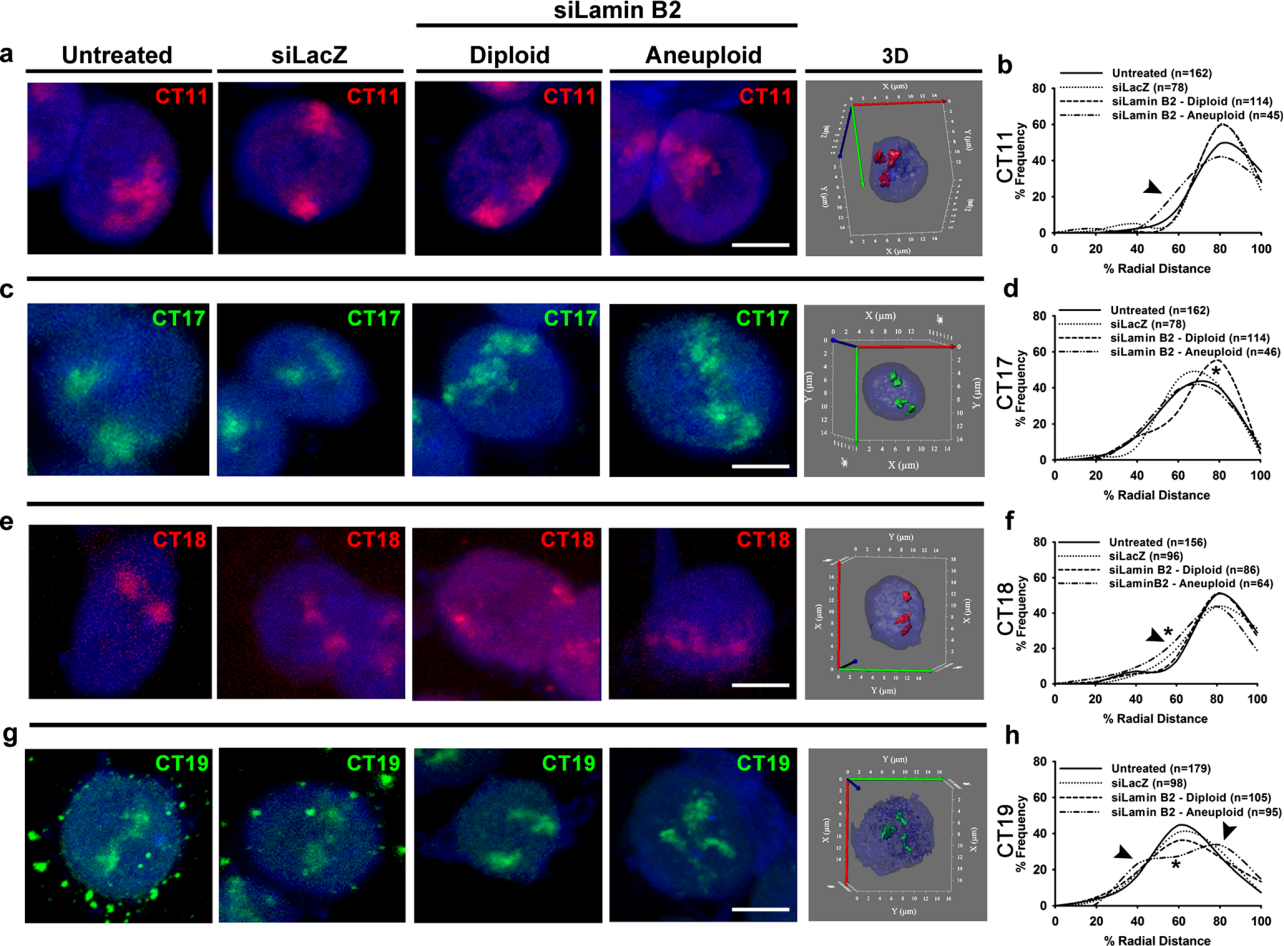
Aneuploid chromosome territories are mislocalized upon Lamin B2 depletion. Images from merged maximum intensity projection of 3D-FISH and radial distance distribution profiles for CT11 (a, b), CT17 (c, d), CT18 (e, f), and CT19 (g, h) which show aneuploidy upon Lamin B2 Kd. Representative 3D reconstructions show aneuploidy in the interphase nucleus upon Lamin B2 Kd. b, d, f, h Radial distance distribution profiles (pooled across experiments) of CT11 (*N* = 2), CT17 (*N* = 2), CT18 (*N* = 2), and CT19 (*N* = 3) binned into five sub-shells of ~20 % radial distance each. In each replicate, radial distances were measured for ~50–100 CTs. b Aneuploid CT11 shows an increase in the sub-population in shell III (RD ~60 %) over the control. d Diploid CT17 shows an increase in the sub-population in shell IV (RD ~80 %) over the control (*p* = 0.0145). f Aneuploid CT18 shows an increase in the sub-population in shell III (RD ~60 %) over the control (*p* = 0.0431). h Aneuploid CT19 showed a significantly different distribution of CT positions as compared to untreated cells (*p* = 0.0157). Specifically, it shows a decrease in the sub-population in shell III (RD ~60 %) over the control (*p* = 0.0061) and an increase in the subpopulations in shell II (RD ~40 %) and shell IV (RD ~80 %), respectively. *Scale bar* ~5 μm. Fisher’s exact and χ^2 ^test was used to test statistical significance of distribution in sub-shells of the nucleus. *N* number of independent experiments (biological replicates) contributing to the data, *n* number of chromosome territories. *Arrowheads*: mislocalized sub-populations of aneuploid chromosome territories upon Lamin B2 Kd
